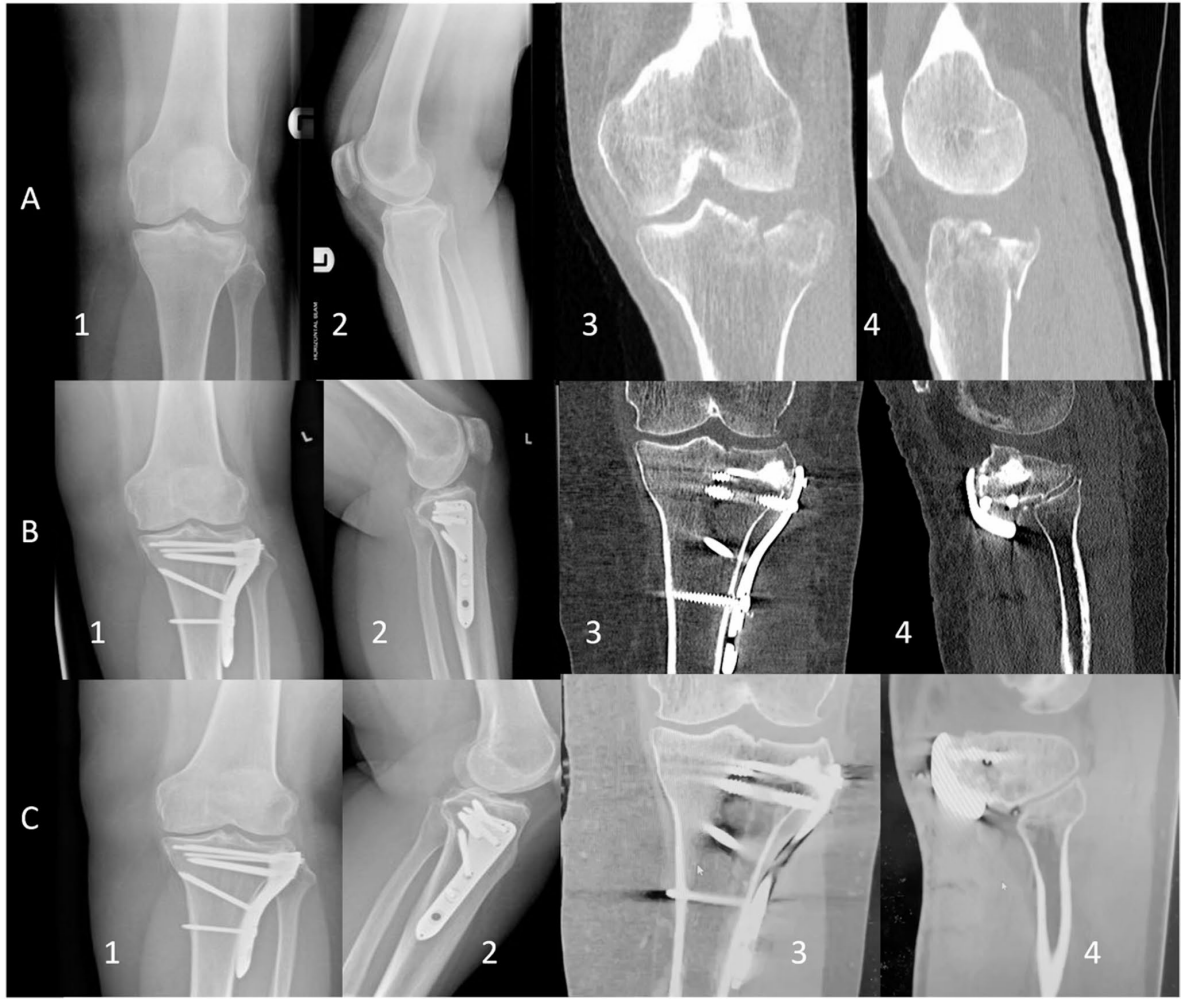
Female 54yoa, Schatzker III or AO/ASIF 41B2 fracture with comminution and depression of the lateral condyle joint surface was managed with an anterolateral surgical approach and plate fixation with joint surface rafting with screws and augmentation with the KUR-111 high bone graft substitute (clearly visible at B3 and B4 CT scan slices). Row A includes plain x-rays and CT scan slices on arrival: AP x-ray (A1); lateral x-ray (A2); coronal cut (A4); sagittal cut (A5). Row B includes plain x-rays and CT scan slices immediately postoperatively: AP x-ray (A1); lateral x-ray (A2); coronal cut (A4); sagittal cut (A5). Row C includes plain x-rays and CT scan slices at 12 months postoperatively: AP x-ray (A1); lateral x-ray (A2); coronal cut (A4); sagittal cut (A5). In comparison to immediate postop imaging, there is evidence of complete healing and the absence of secondary collapse at the comminuted lateral tibial plateau joint surface
